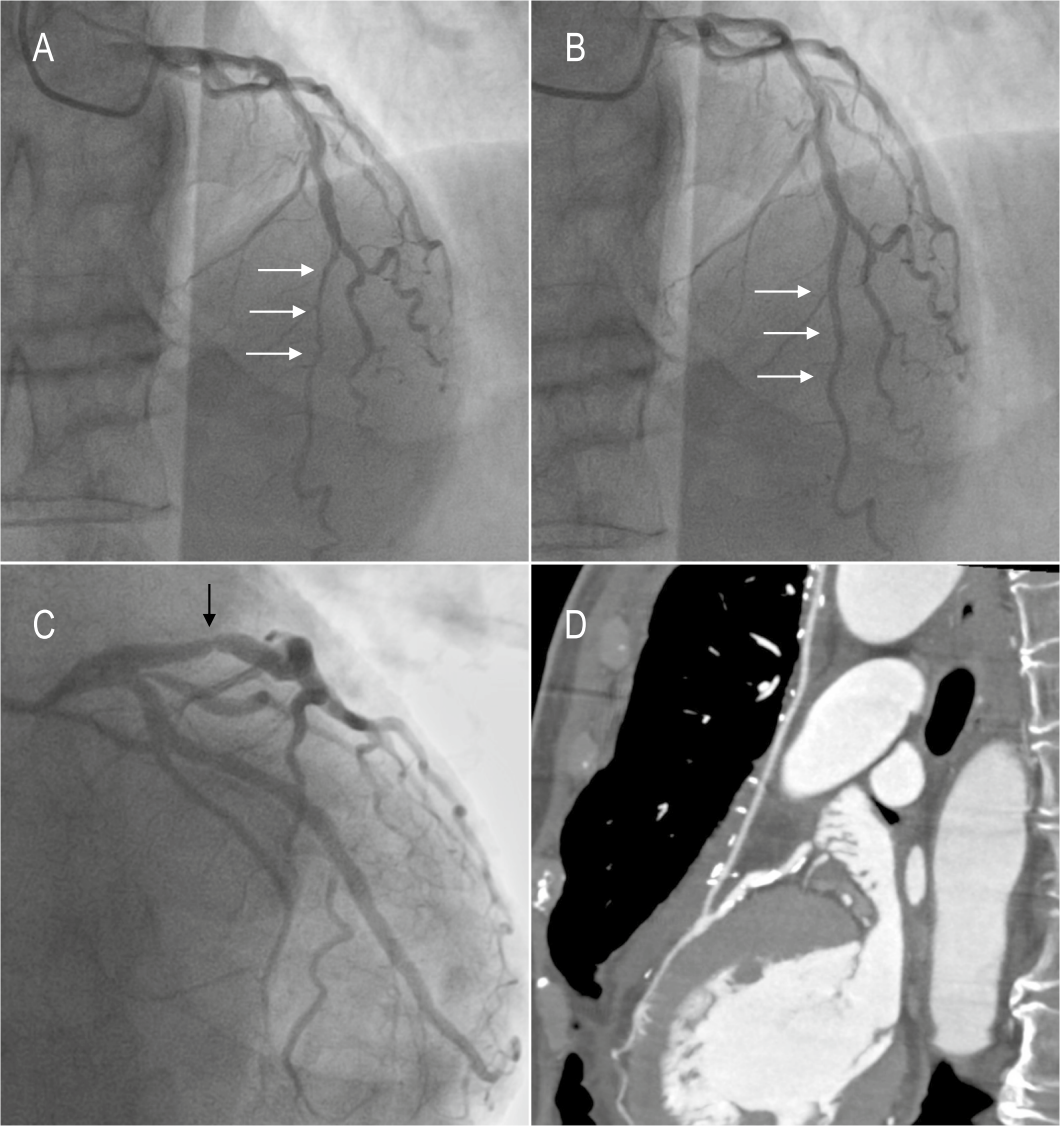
Coronary angiography before surgery and coronary computed tomographic angiography (CCTA) during the follow-up period. Systolic compression of the mid LAD (A, white arrow), complete recovery in the same area in diastole (B, white arrow). A atherosclerotic stenosis of the proximal segment of the left descending branch (C, black arrow). CCTA after surgery demonstrates complete release of MB and graft patency at 6-month follow-up (D)
